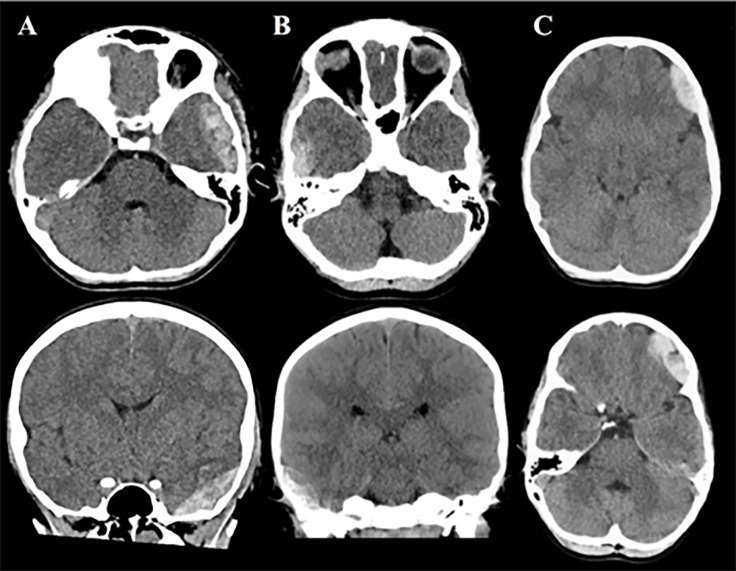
Computed tomography images of three hematomas not detected by the handheld brain scanner.
